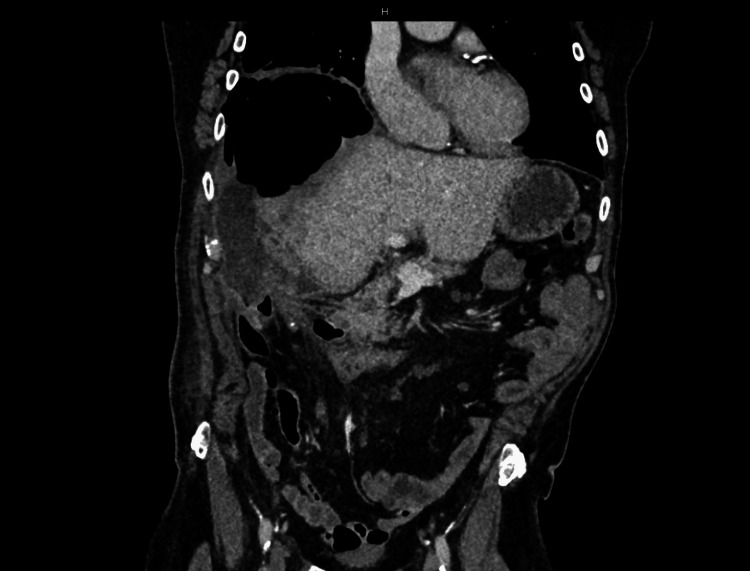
Surveillance CT of the abdomen and pelvis one year post-surgery, showing a subdiaphragmatic collection containing gas pockets. CT: computed tomography.
